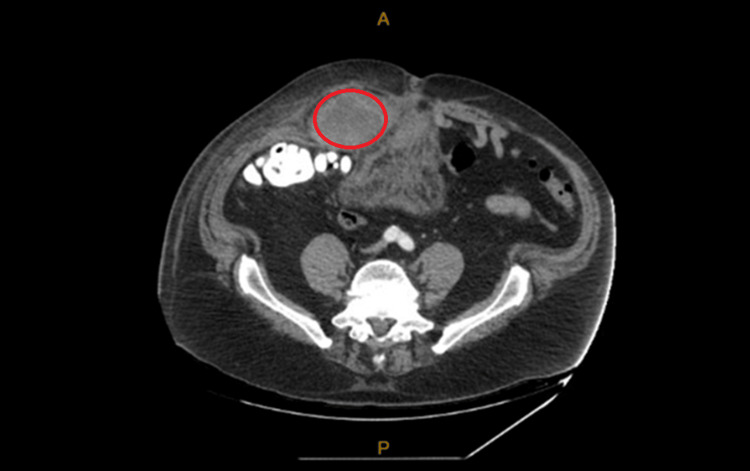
CT of the abdomen with contrast. Red circle showing rectus sheath hematoma measuring 14.6 cm x 8.2 cm.
